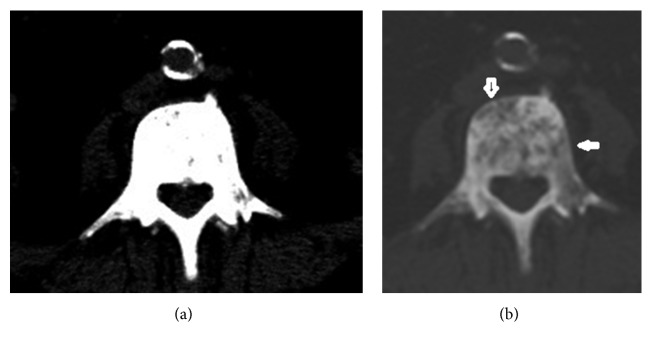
A case of prostate cancer with osteoblastic metastasis. (a, b) CT scan showing a bone window tumor with osteoblastic metastasis spread to anterior and lateral portions of the vertebra. Arrows indicate lesions and spreading routes within the vertebral body.
